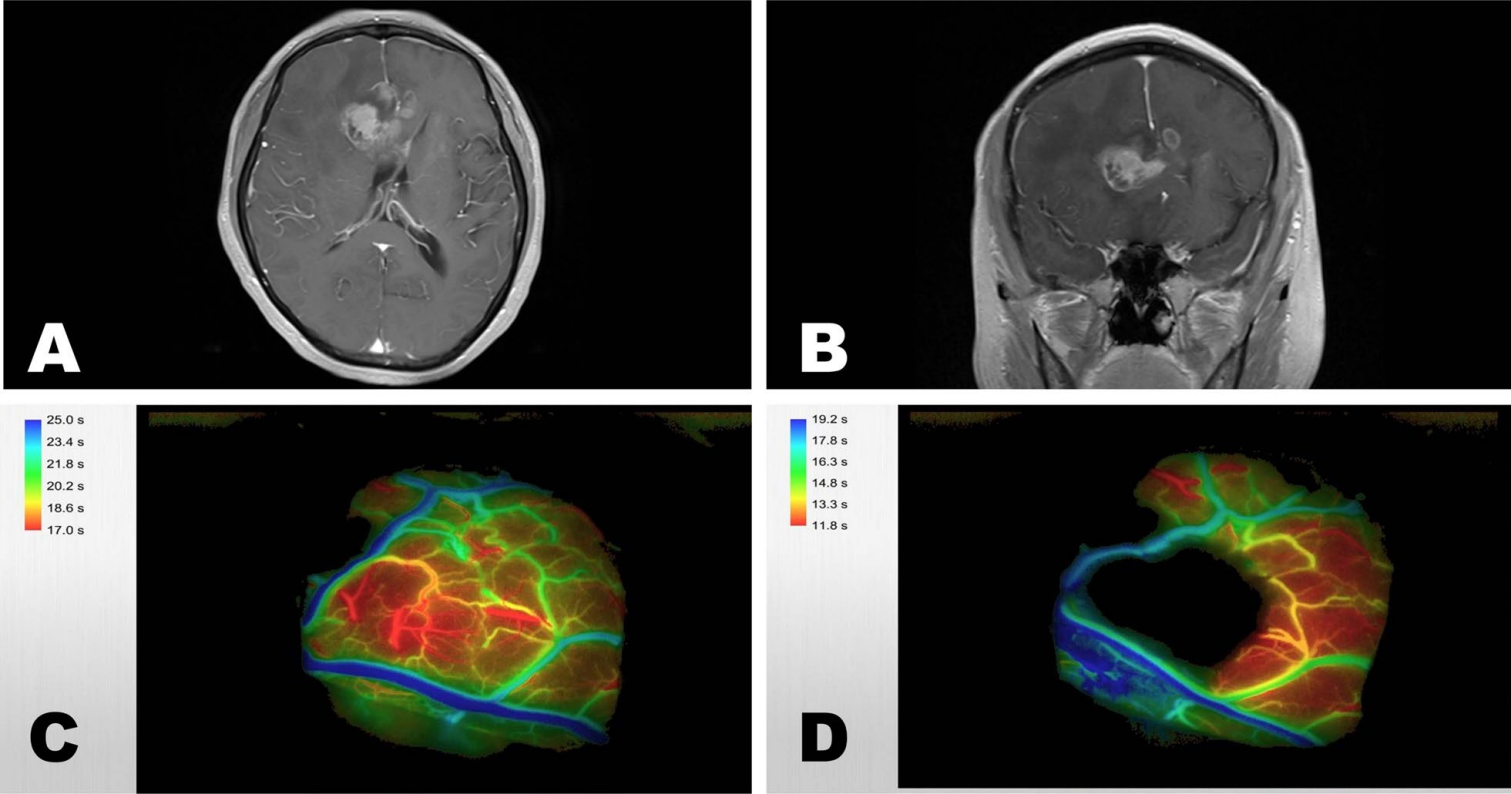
A patient with a mass on the bilateral frontal lobe and corpus callosum. A, B MRI with contrast showed a heterogeneous mass with cystic areas involving the body of the corpus callosum and extending bilaterally into the frontal lobes FLOW 800 was used to map the blood flow conditions of the two superior cerebral veins C before and D after tumour resection
